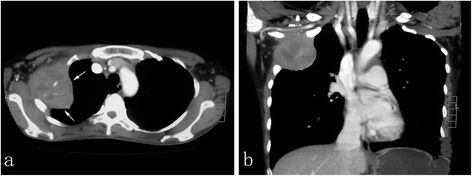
Radiographic examination of the lesion. (a) Osteolytic lesion of the right second rib with associated soft tissue mass appeared to have a distinct border to the lung in periphery in computed tomography scan (white arrow). (b) The osseous destruction of rib showed adjacent soft tissue extensions with moderate gadolinium enhancement but no involvement of regional lymph node was observed.
